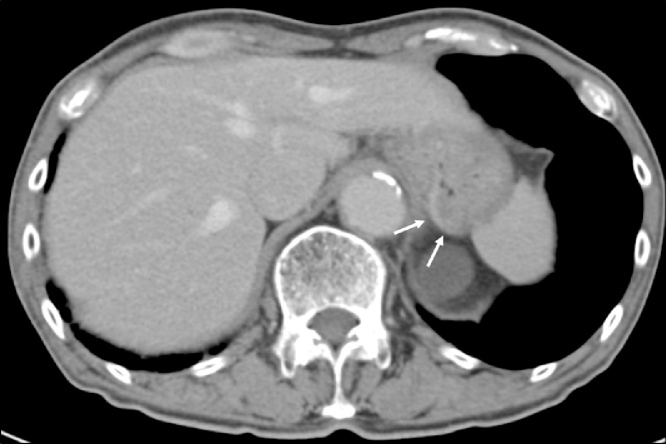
Abdominal computed tomography revealed thickening of the posterior wall of the upper part of the stomach with contrast effect (*arrow*) and no enlarged abdominal lymph nodes or distant metastases.
